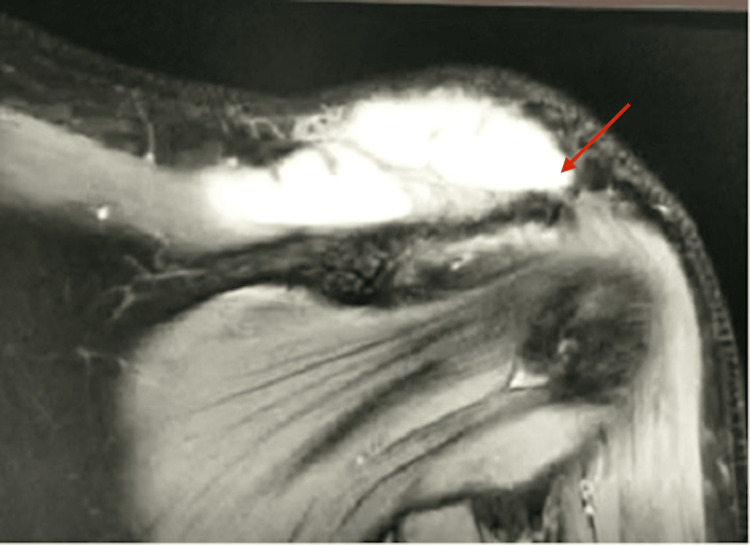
MRI of the left shoulder, showing an irregular collection in the left acromioclavicular joint (arrow represents the area of collection)
